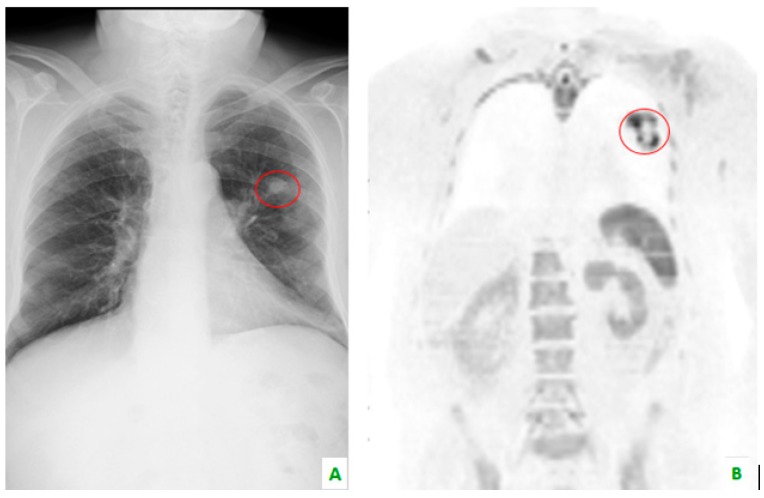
(A) This chest X-ray reveals, in the red circle, a lung tumor which is also visible in (B) whole body magnetic resonance imaging with diffusion-weighted imaging (WB-MRI-DWI) in the coronal plane, in the red circle.
